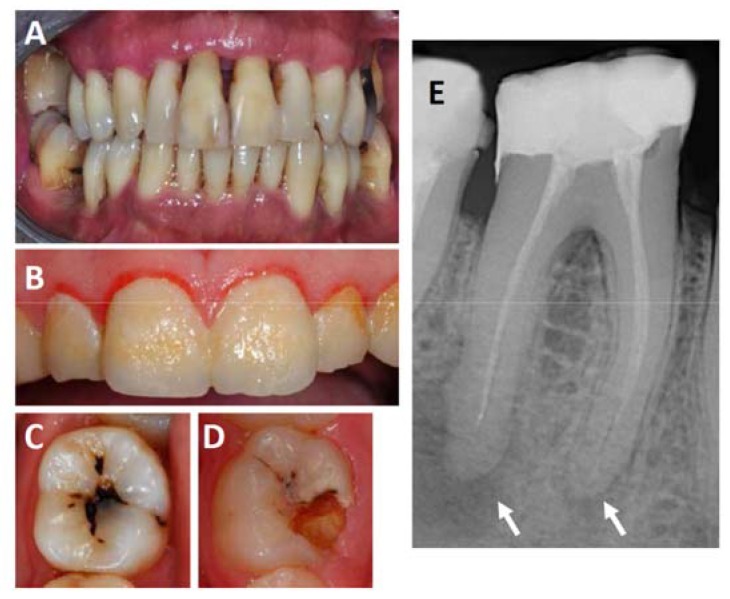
Frequent oral inflammations affecting the teeth, gingiva and the periodontium. (A) Shows an example of a severe case of periodontitis. Note the extensive loss of attachment and gingiva recession visible at most teeth; (B) shows a case of gingivitis. Note the soft plaque that covers the entire surface of the teeth and the gingiva reddened by the inflammation; panels (C,D) show examples of teeth affected by root caries, which often lead to the formation of endodontic lesions in the form of periapical abscesses, which can be detected on radiographs as shown in panel (E). Arrows mark the locations of periapical abscesses.
